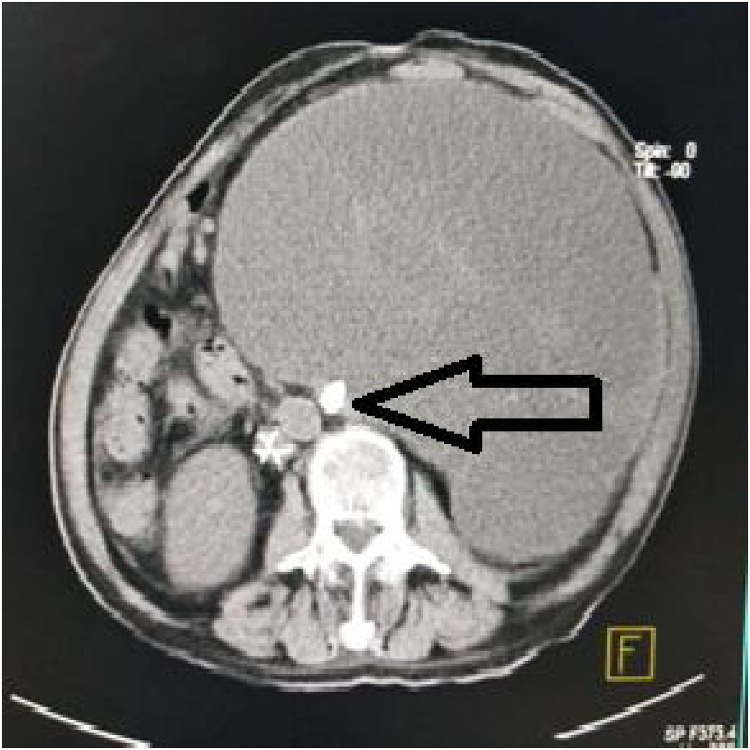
CT scan of the abdomen (transverse section) showing GH, and a high-density shadow (black arrow) located in the renal hilum region.
